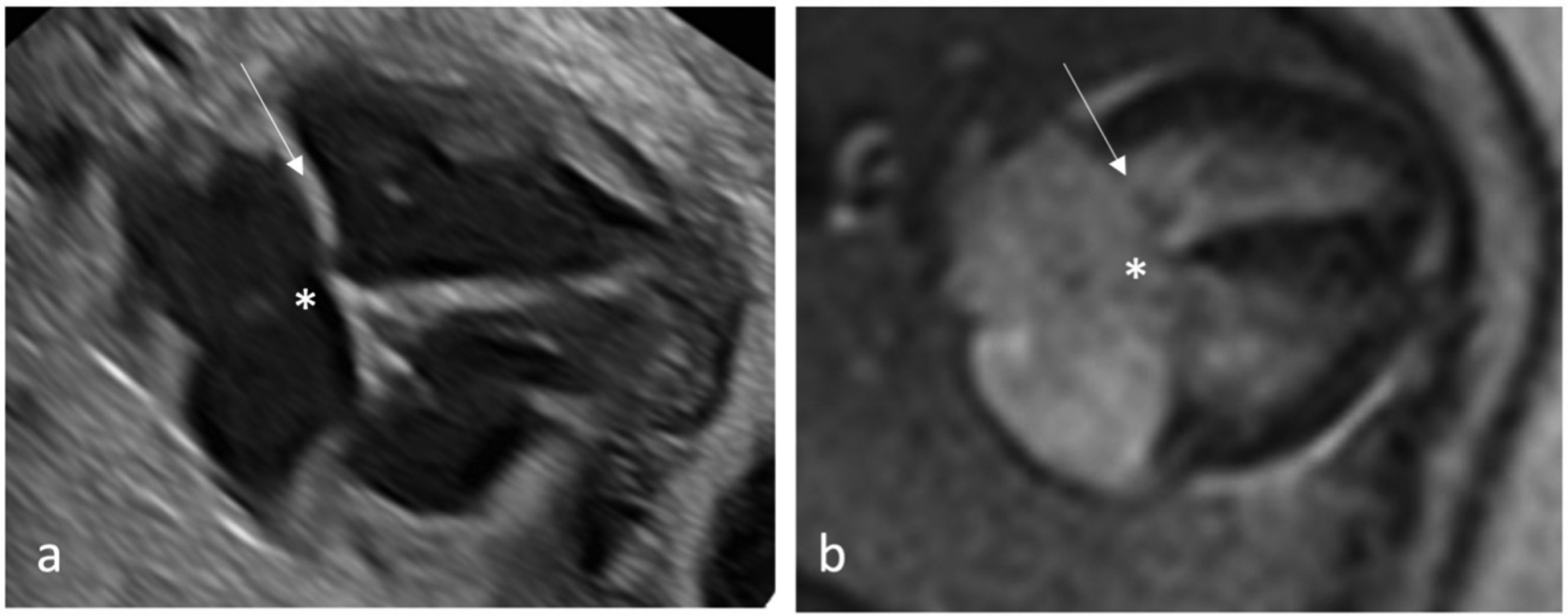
Qualitative assessment of fetal cardiac 4CV on fetal echocardiography (a) and DUS gated fetal cardiac MRI (b) with intermediate type AVSD detected by both methods. An AVSD, as shown here, is an atrial septal primum defect and an inlet ventricular septal defect (asterisk) with a combination of an abnormal common atrioventricular valve with a linear insertion (arrow).
